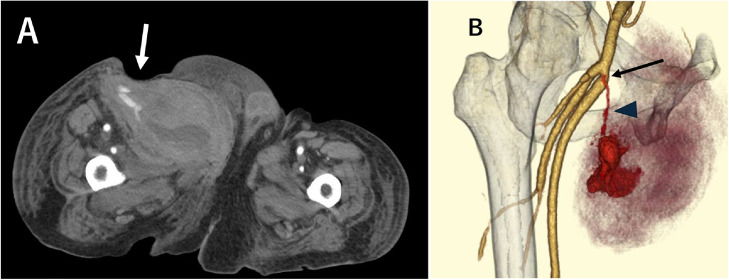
Initial imaging of a femoral artery pseudoaneurysm. (A) Contrast-enhanced computed tomography scan demonstrates a large femoral artery pseudoaneurysm with active contrast extravasation (arrow). (B) Three-dimensional, volume-rendered image of the pseudoaneurysm arising from a long arterial sheath puncture tract (arrowhead). The tract origin is indicated by an arrow near the bifurcation of the superficial and deep femoral arteries.
